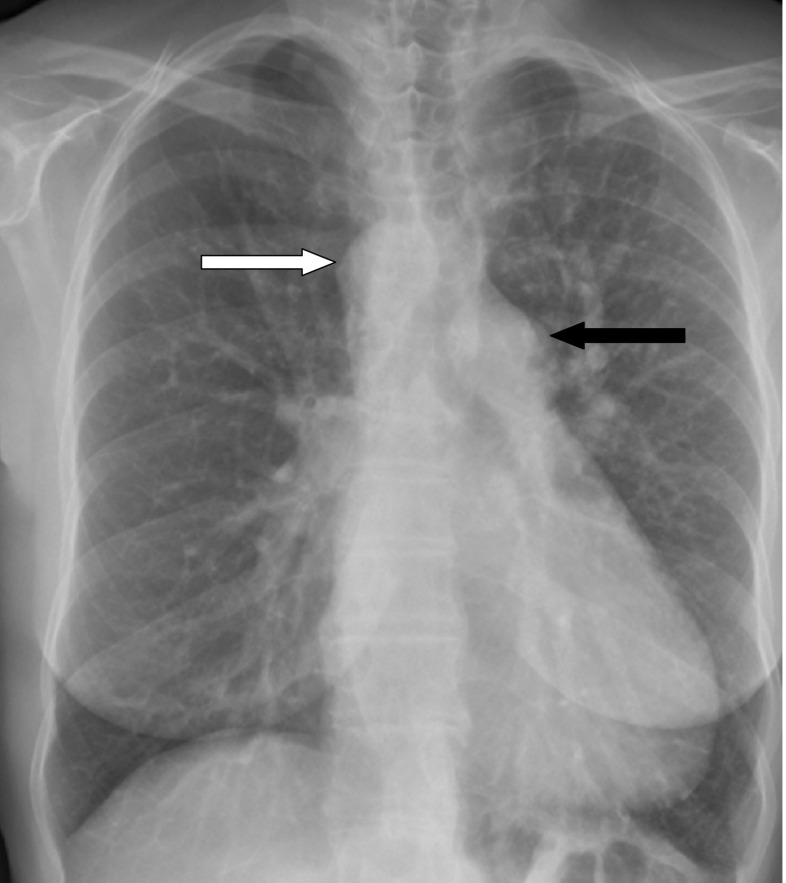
Chest radiography showing an enlarged heart figure, a right descending aorta (white arrow) and an enlarged left pulmonary artery (*black arrow*)
